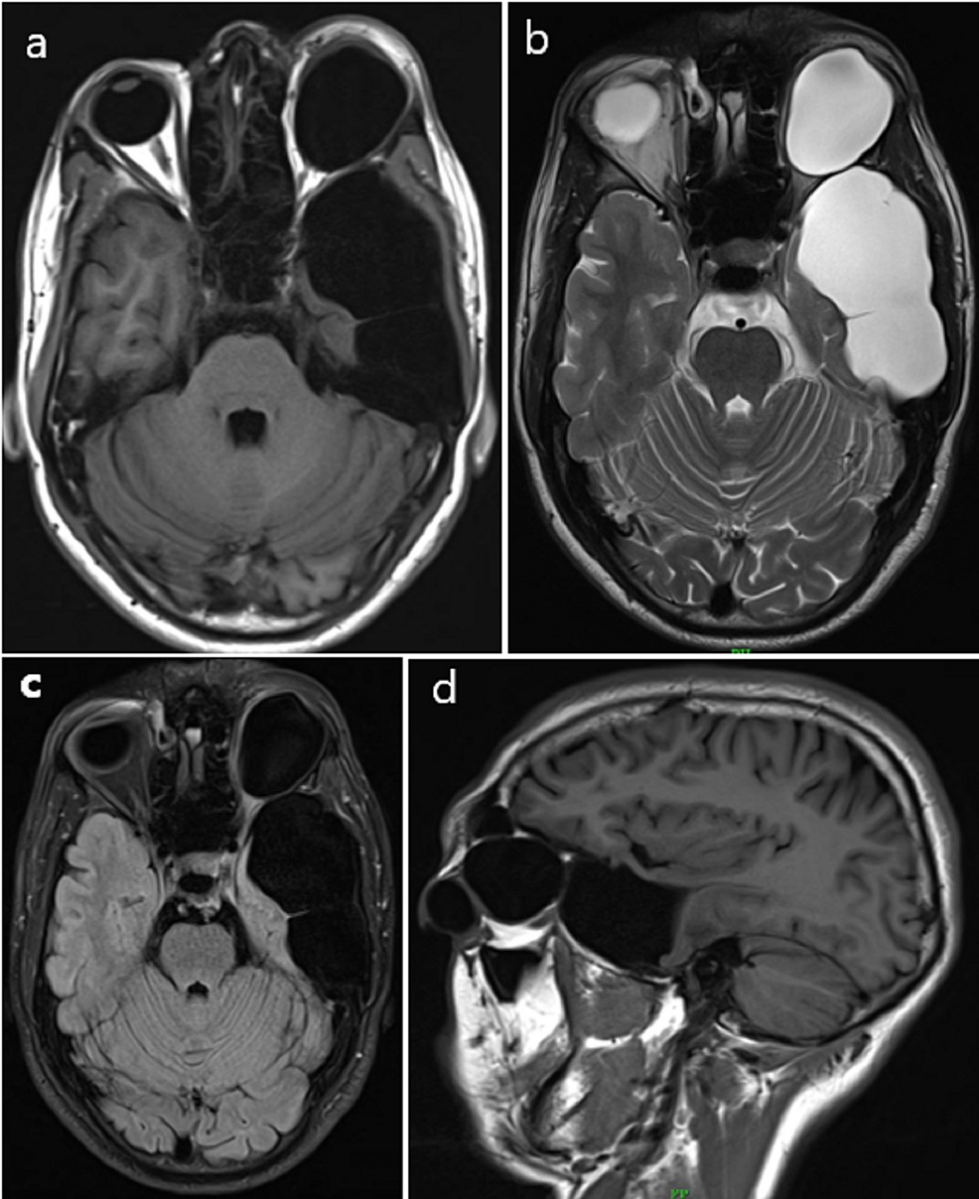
(a) Axial T1WI. (b) Axial T2WI. (c) FLAIR axial. (d) Sagittal T1 images showing CSF signal intensity extra-axial cystic lesion in the left middle cranial fossa and intraorbital cystic lesion.
